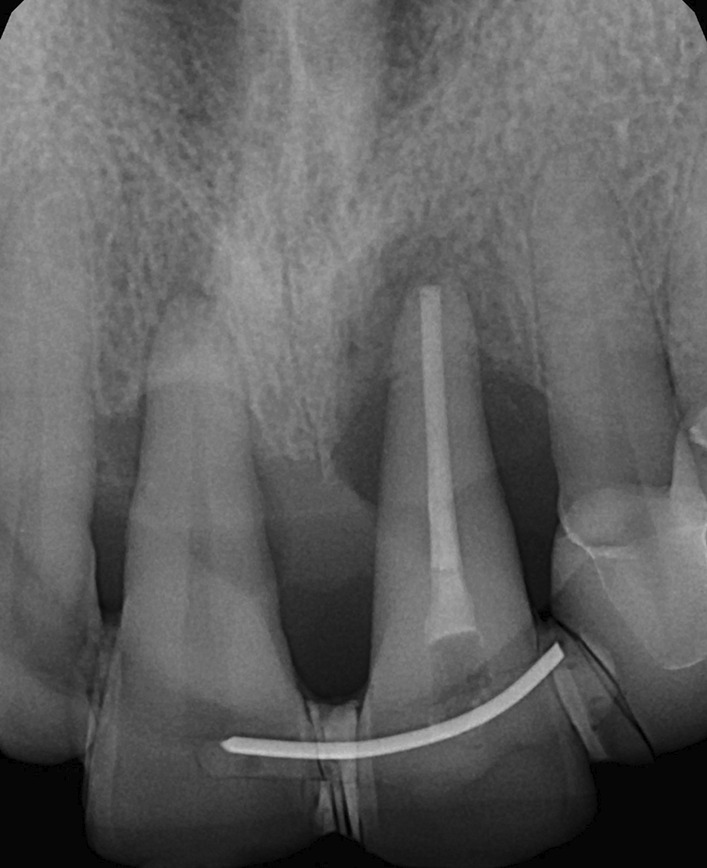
Baseline intraoral X-ray
